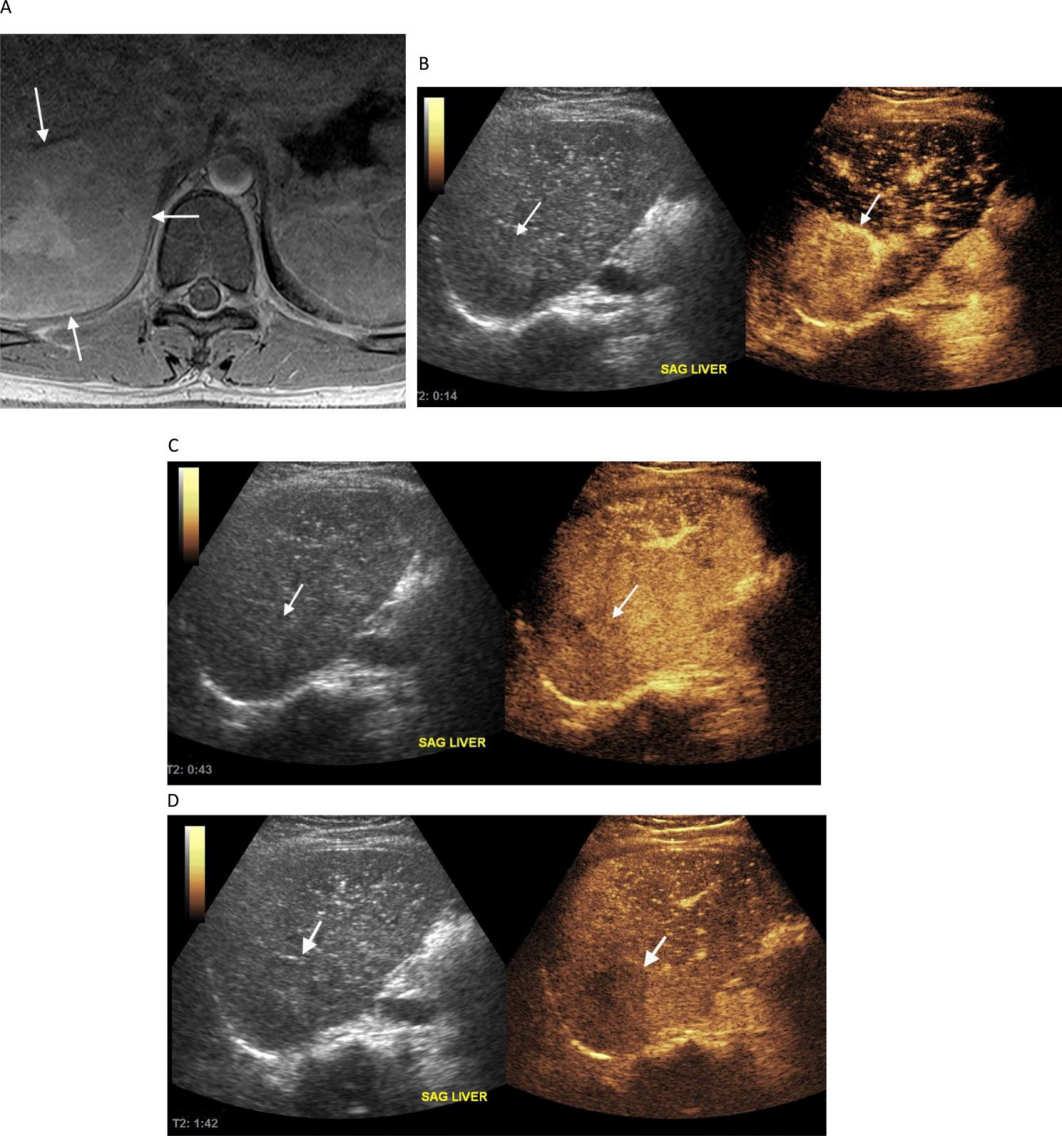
18-year-old male, medulloblastoma survivor, 12.5 years after completion of therapy, developed a focal liver lesion (FLL) that was incidentally discovered as (A) heterogeneity within the liver (arrows) on T1W contrast enhanced axial spine MRI. (B) Arterial-phase contrast enhanced ultrasound (CEUS) image obtained 14 s after contrast administration shows hyperenhancement of the lesion (arrows). (C) Portal-venous-phase CEUS image obtained at 43 s and (D) delayed-phase CEUS image obtained at 1 min 42 s after injection show hypoenhancement of the lesion. This was pathologically proved to be focal nodular hyperplasia
